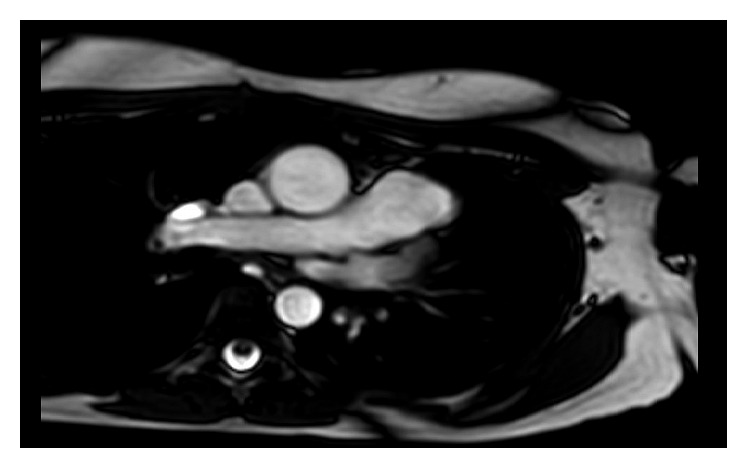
MRI cardiac showing interposition of lung tissue between aorta and pulmonary artery.
